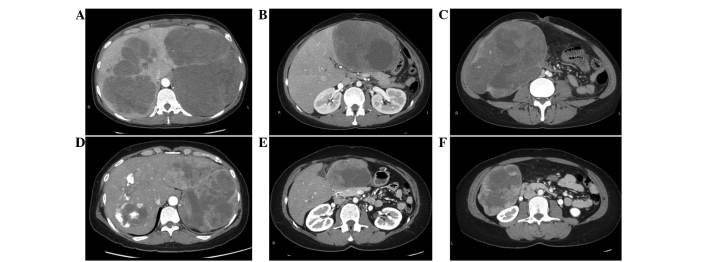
Abdominal CT images pre- and post-chemotherapy. (A) Abdominal CT showed recurrence of the local tumor following distal pancreatectomy in the form of a large tumor (15 cm in diameter) in the left subphrenic area, as well as liver metastases in the left lateral segment and anteroposterior segment. (B) The left lateral segment was occupied by a tumor, which was palpable in the upper abdomen. (C) A large mass of the lower posterior segment was palpable in the right lower quadrant of the abdomen. (D–F) Following combination therapy with gemcitabine hepatic arterial infusion, plus oral S-1 and transarterial embolization of the anteroposterior segment, the recurrent local tumor was slightly reduced in size and the liver metastases were obviously reduced. CT, computed tomography.
